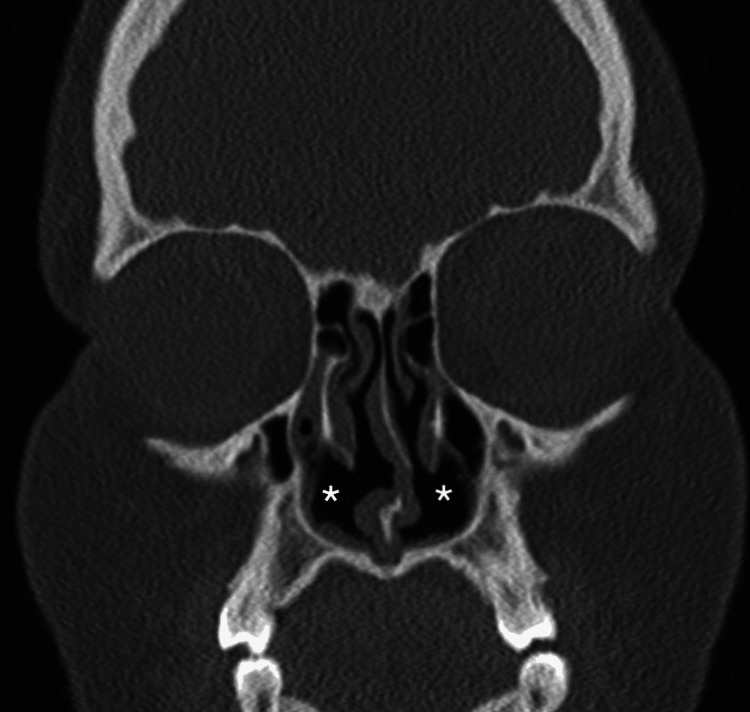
Coronal view CT scan of the sinus showing the bilateral agenesis of the head of the inferior turbinates The asterisk represents the agenesis of the head inferior turbinate. CT: computed tomography
